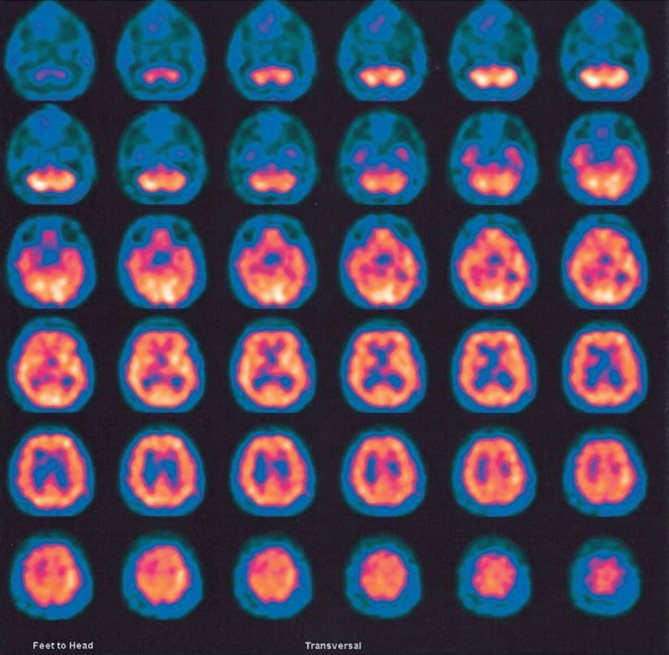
DAT SPECT imaging showing reduced radiotracer uptake in the basal
